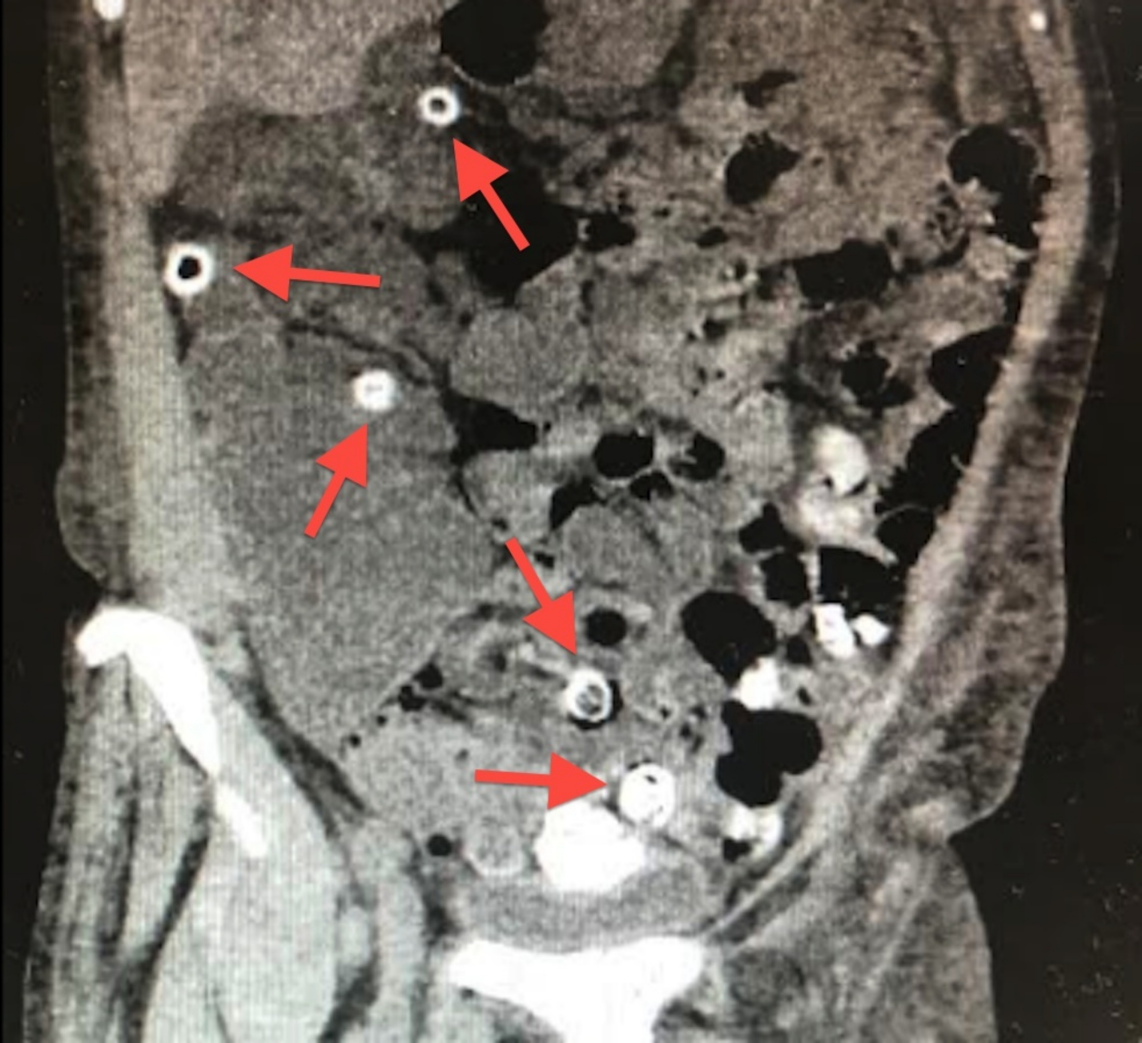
A coronal non-contrast computed tomography (CT) of calcified diverticula throughout the colon causing rectal bleeding
